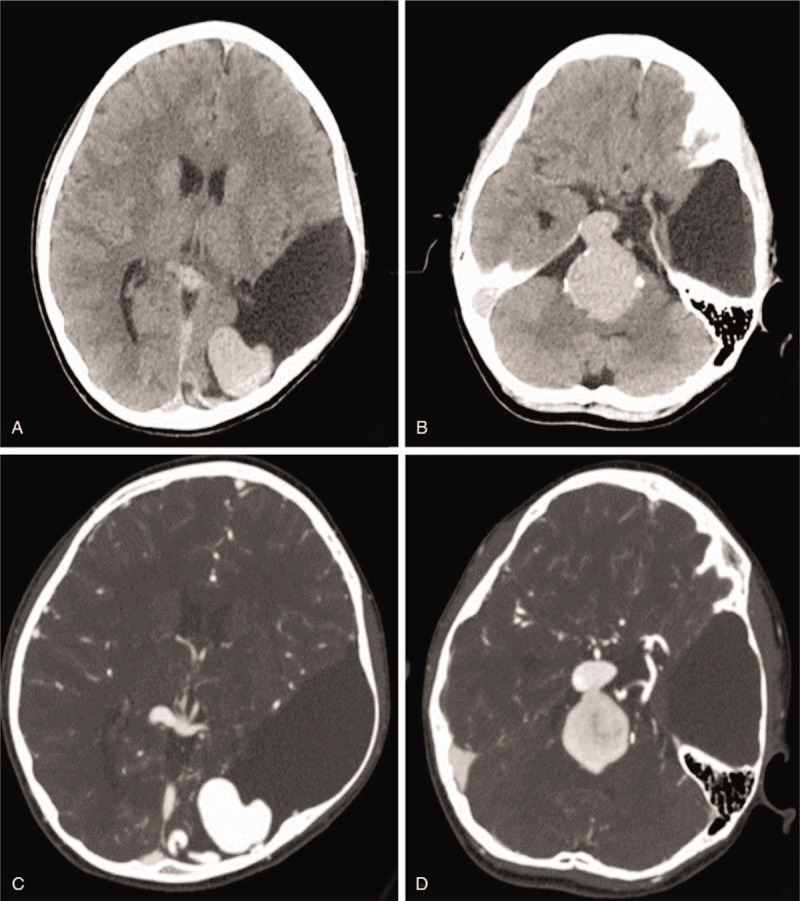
Computed tomography (CT) scan images showing the bilateral pial arteriovenous fistulas accompanying a giant arachnoid cyst in the left occipital and temporal region. (A, B) Two slightly high-density masses at the anterior pontine cistern, right circumferential cistern, and left posterior occipital region. (C, D) Enhanced CT scan showing the degree of vascular enhancement.
